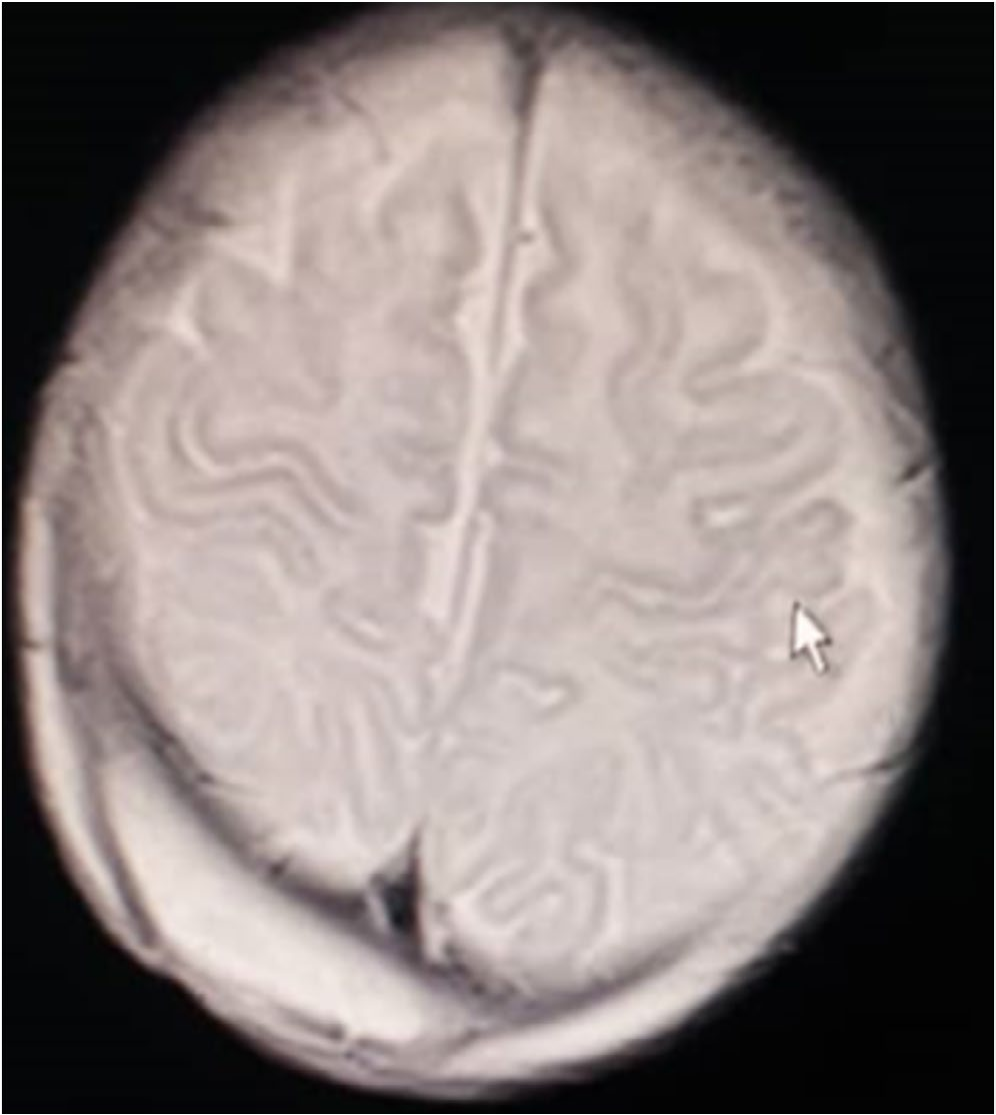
Brain MRI of head demonstrating a large collection in the right occipital region.
